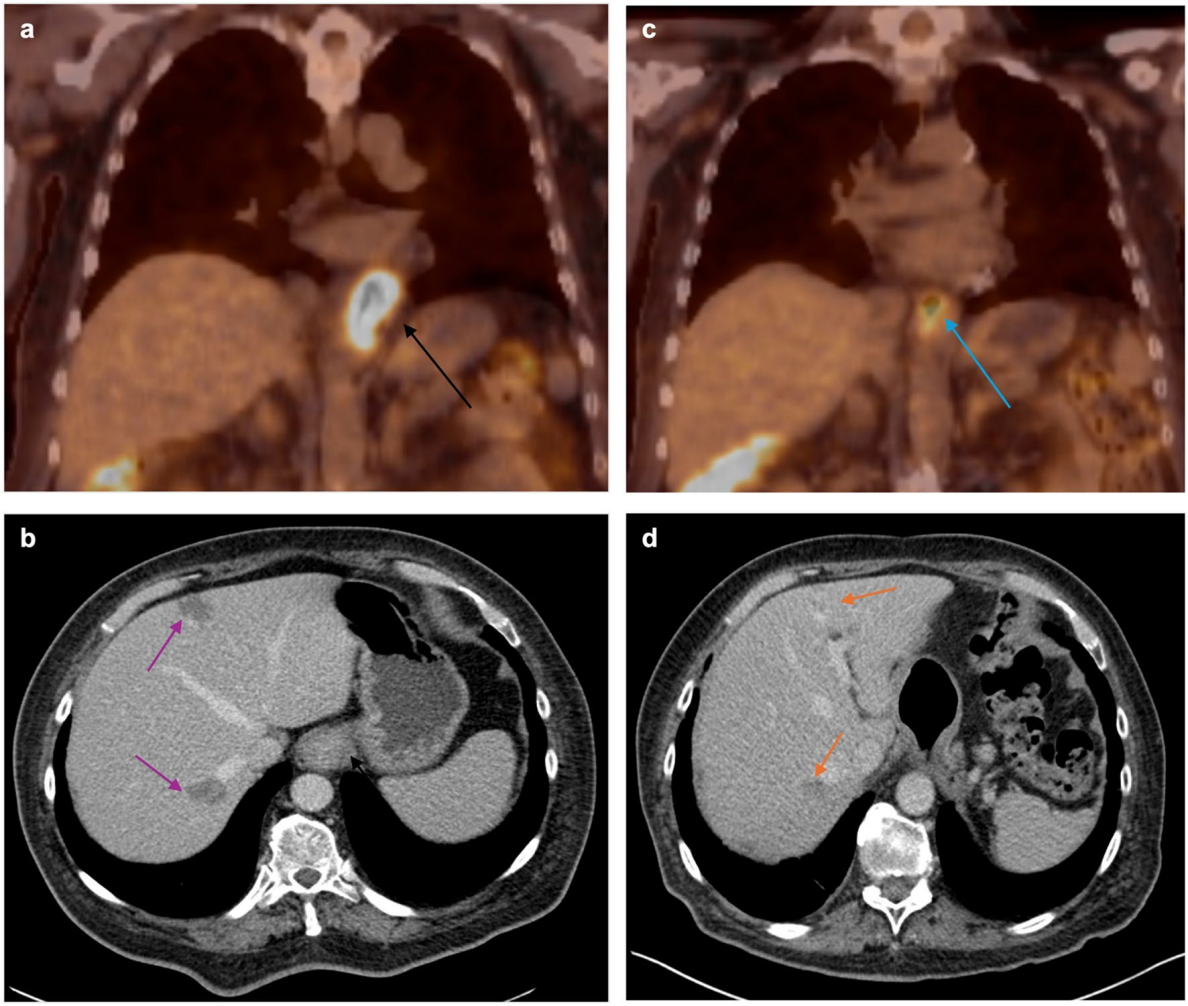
FDG PET/CT of a distal esophageal adenocarcinoma at the time of initial staging (a) demonstrating a high degree of PET avidity with a max SUV of 12.7 in addition to a CT (b) showing a circumferential mass of the distal esophagus (black arrow) and hepatic metastases (purple arrows). FDG PET/CT after 2 months (c) of chemoradiation with Taxol and carboplatin showing significantly reduced metabolic activity with a max SUV of 4.2 (blue arrow). Three and a half years after esophagectomy and initiation of nivolumab, CT (d) shows treated hepatic metastases (orange arrows) without evidence of active disease
